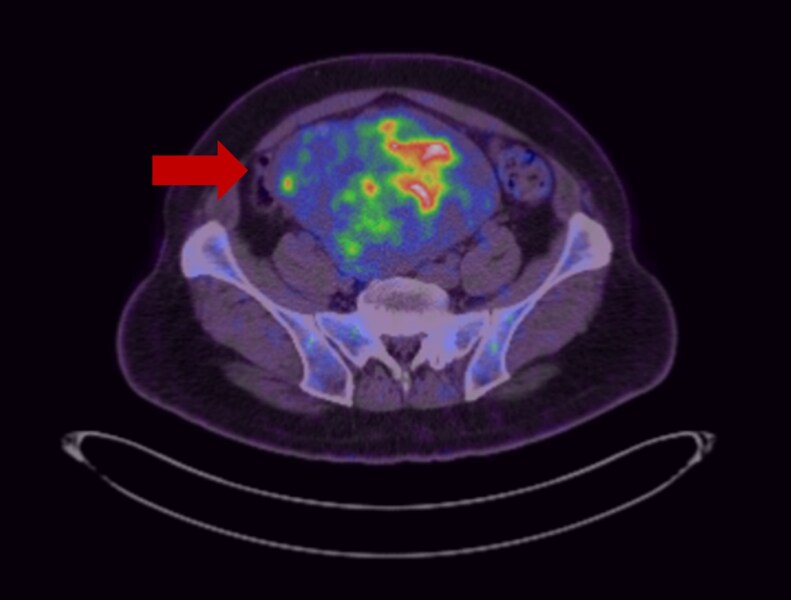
^18^F-Fluorodeoxyglucose positron emission tomography (FDG PET) image demonstrating the heterogenous FDG avidity in the lower abdominal mass (arrow).
